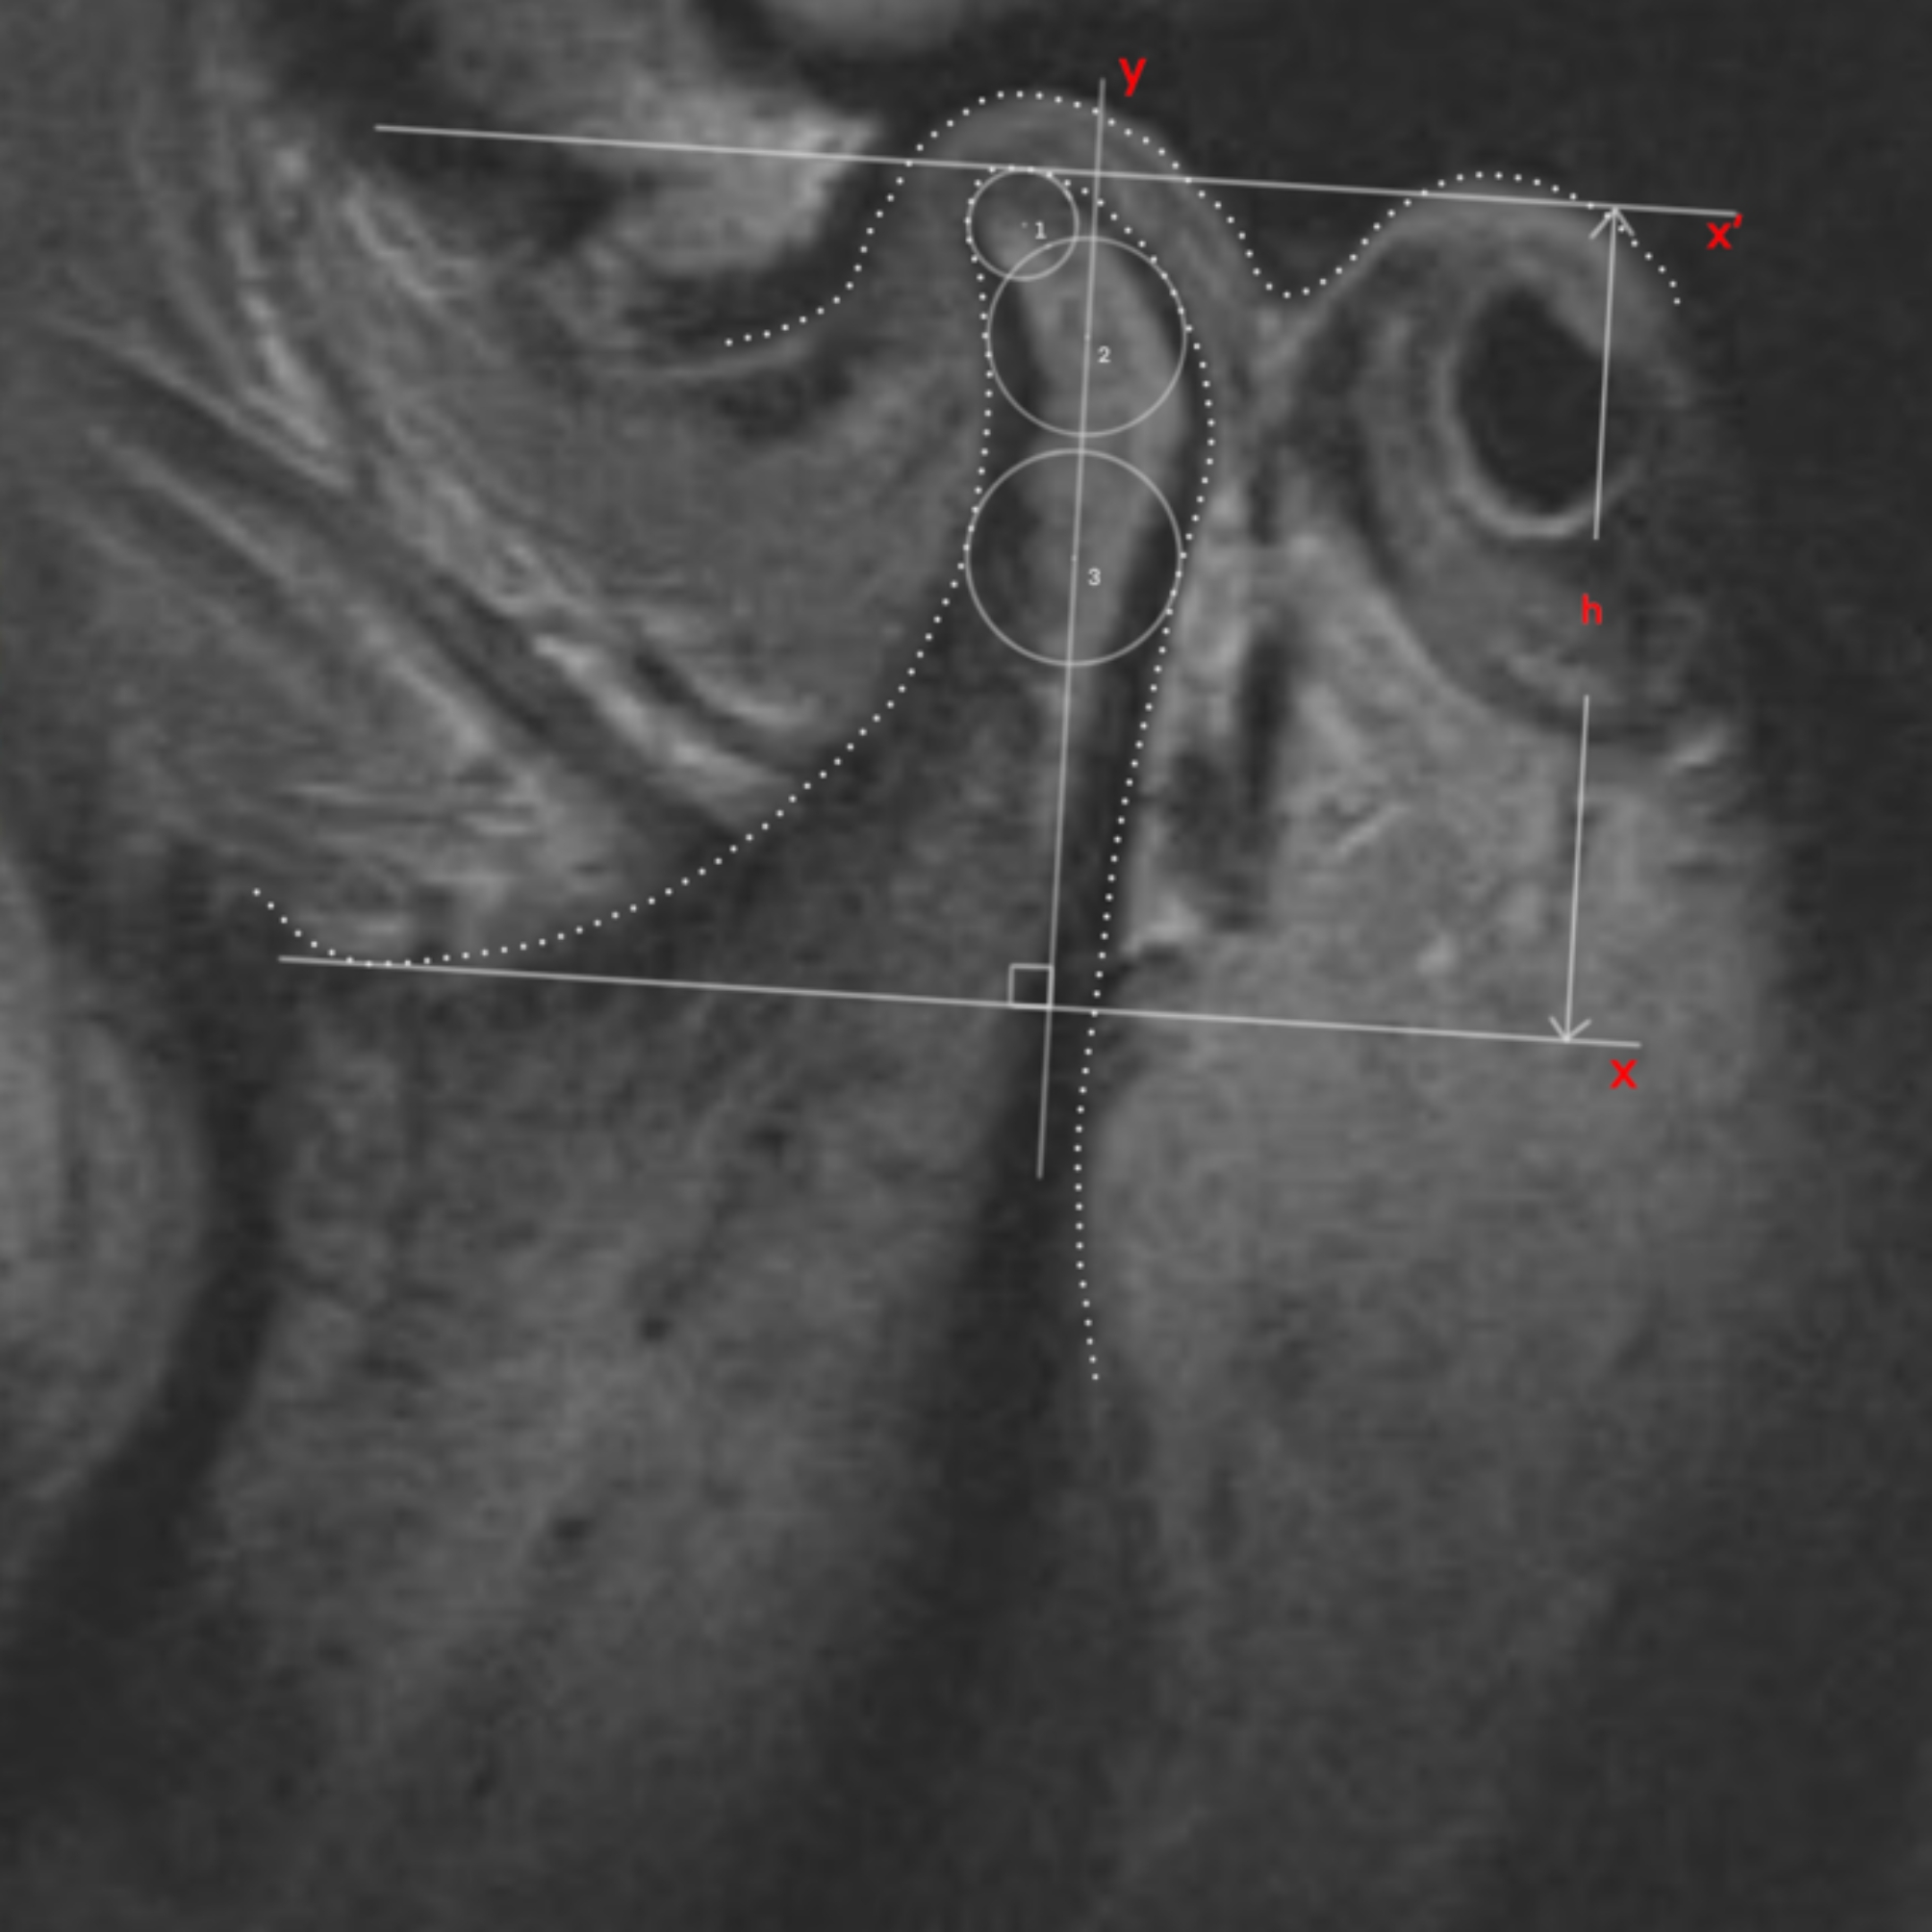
The condylar height measured by three-circle method in MRI
